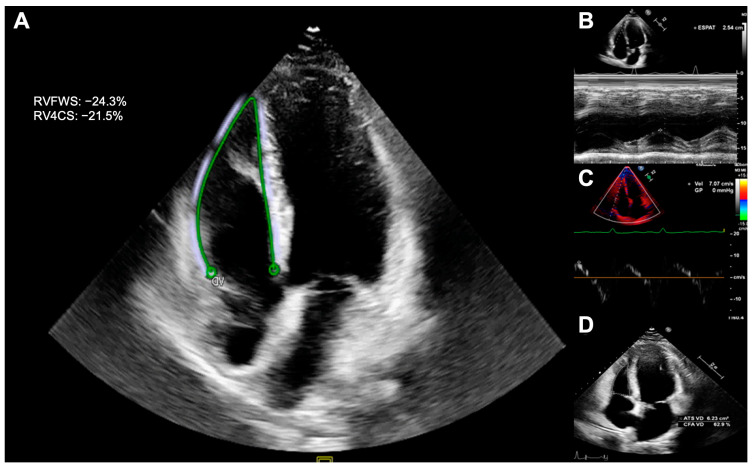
Echocardiographic assessment of right ventricular systolic function. Right ventricular strain (A), tricuspid annular plane systolic excursion (B), s’DTI (C), and fractional area change (D).
